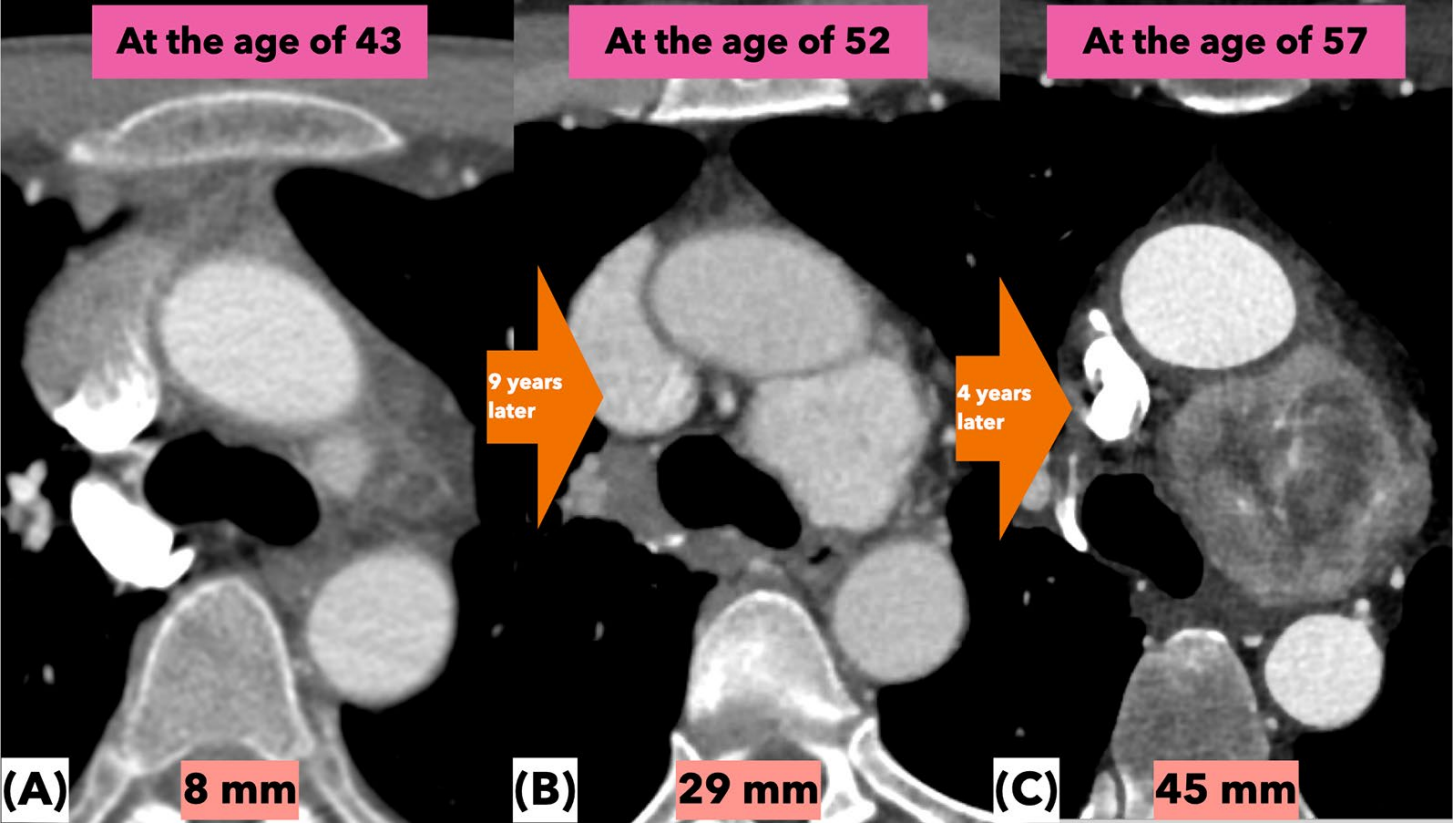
Computed tomographic images depicting serial tumor growth. When the patient was 43 years old, the tumor measured 8 mm in diameter and subsequently exhibited a gradual tendency to expand (A). Nine years later, the tumor size increased up to 29 mm (B) and it turned out to be 45 mm 4 years later (C). As the tumor expanded, the compression of the left bronchus and esophagus progressed over time
